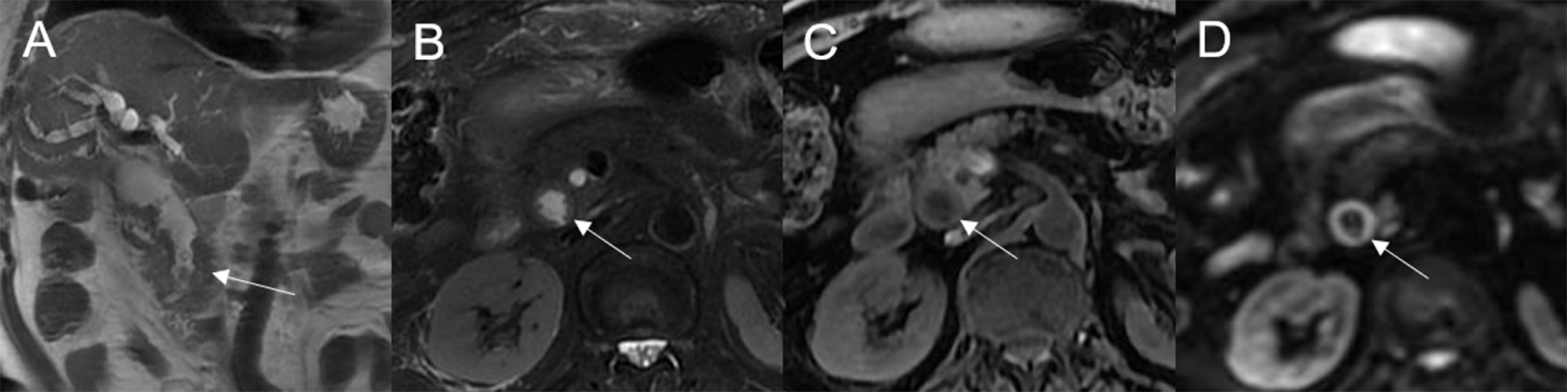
Adenoma in the common bile duct. The plaque-like mass (white arrow) shows isointensity on T2WI (A, B), isointensity on T1WI (C), and hyperintensity on DWI (D). The bile duct lumen at the lesion site is narrow and the diameter of the bile duct is enlarged. Coronal T2WI shows dilatation of the bile duct upstream of the lesion (A).
